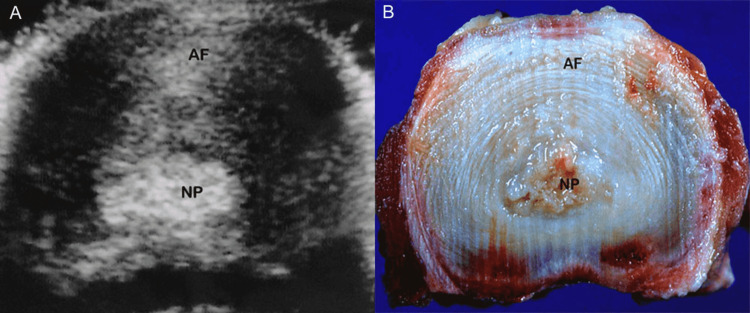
Normal disc, ultrasound. (A) Axial high-resolution sonographic image outlines the hyperechogenic nucleus pulposus (NP). There are numerous fine echoes in the annulus fibrosus (AF) at the periphery of the disc. (B) Corresponding anatomic section in axial plane delineates nucleus pulposus (NP) engulfed by annulus fibrosus (AF).
